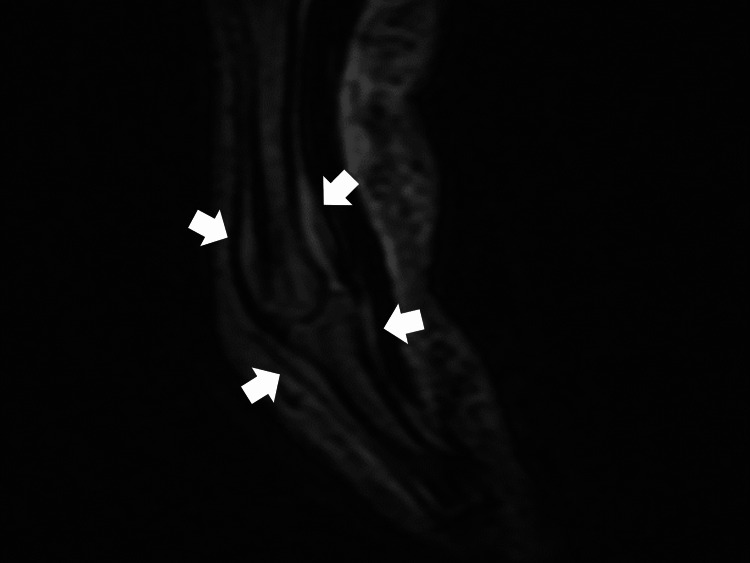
Magnetic resonance imaging of the right hand (short Tau inversion recovery views) showing high-intensity signal areas around the joint, such as at the tendon attachments (white arrows).
